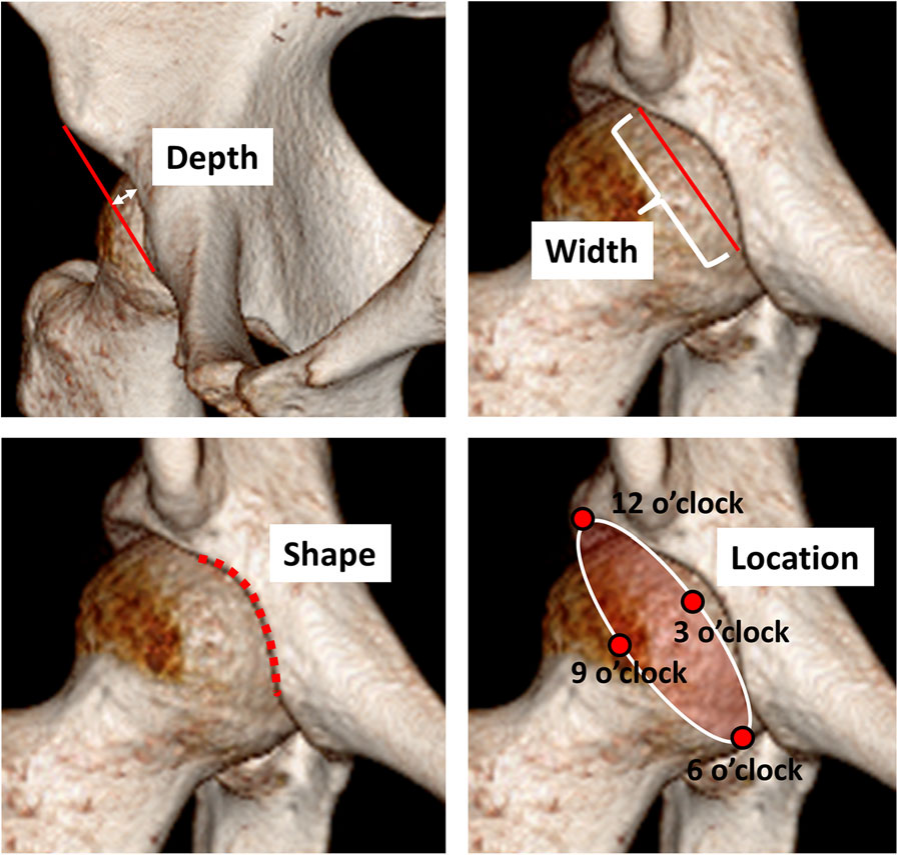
Dimensions of the psoas valley in 3D-CT scan
